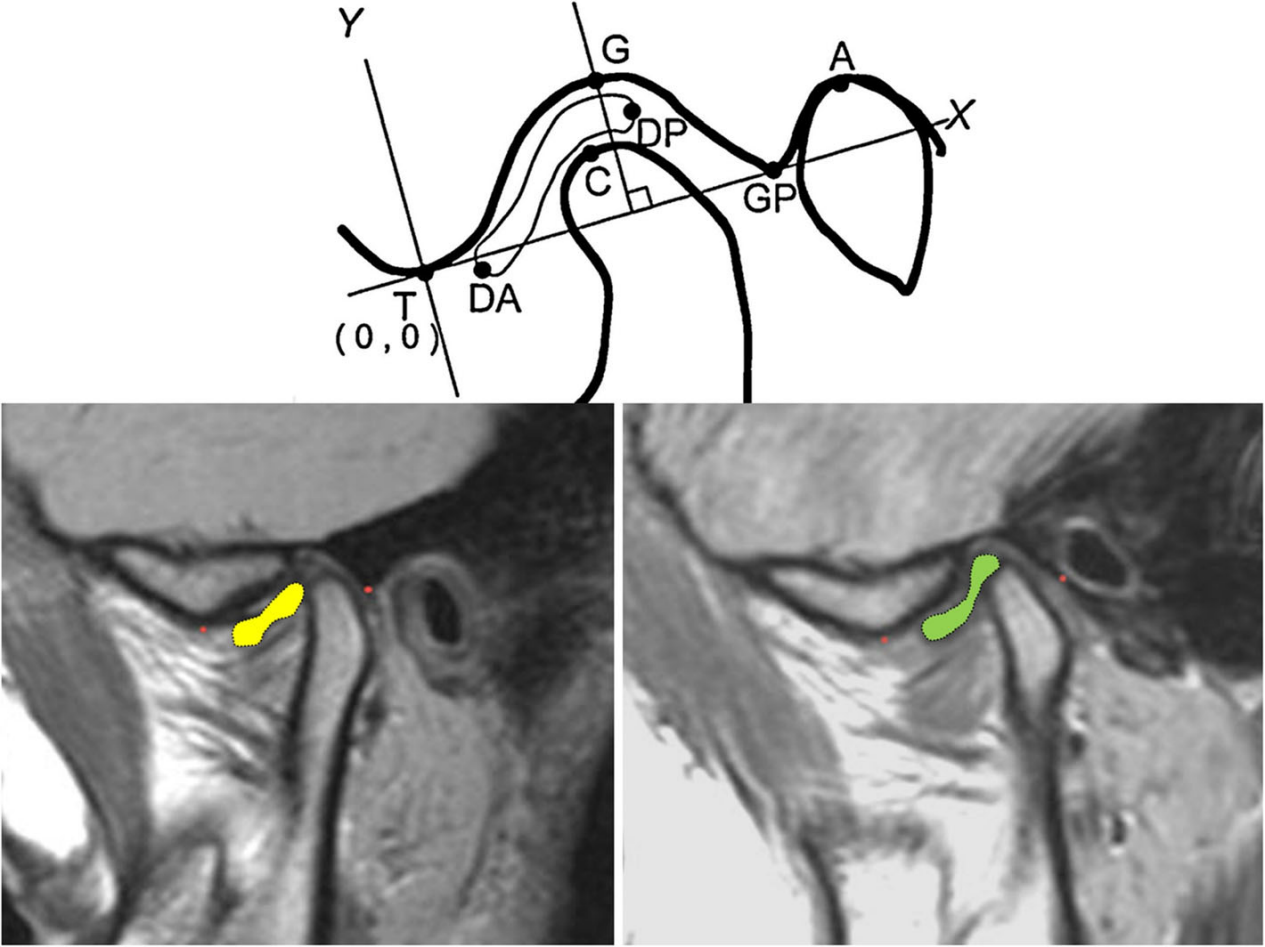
Image showing a graphical representation of the point system used to locate the articular disc on the MRI (adapted from Arayasantiparb et al. [1] [top]). MRIs of one of our patients, preoperative left and postoperative left. Red points identify the T (lowest point of eminence will mark (0,0) on the (*x*,*y*) graph) and GP (posterior glenoid tubercle) (will mark (10,0) point of the (*x*,*y*) graph to be drawn). The discs are coloured for visualization [bottom]. Point DA, anterior-most convexity of the articular disc; point DP, posterior most convexity of the articular disc; point C, uppermost point of the mandibular condyle; point GP, lower-most point of the posterior glenoid tubercle; point A, uppermost point of the external auditory meatus
